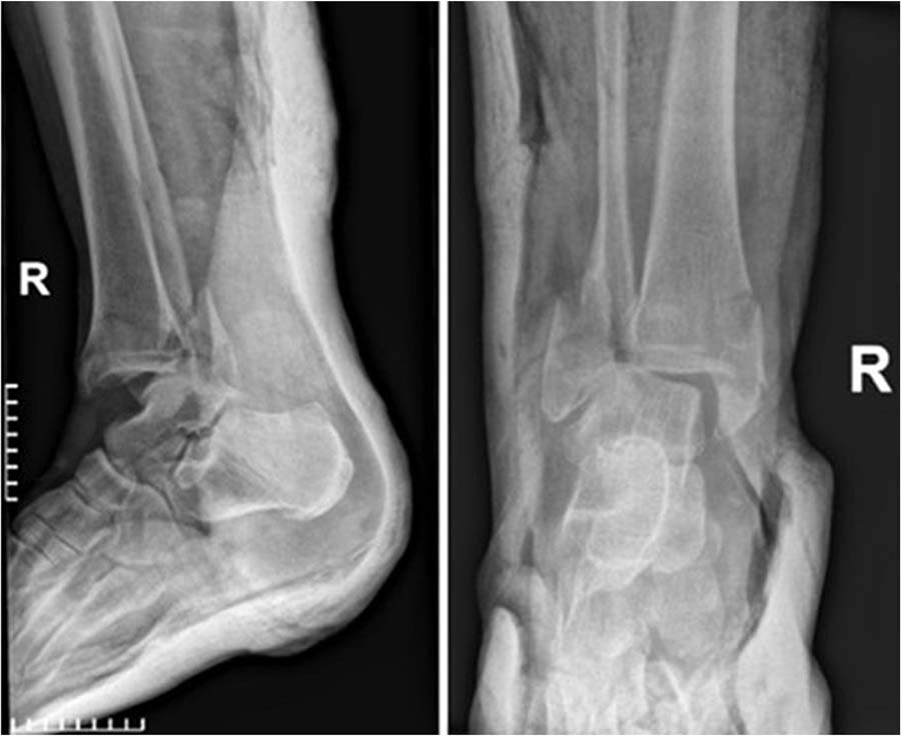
Anteroposterior and lateral X-ray of the ankle joint showing the trimalleolar fracture.
